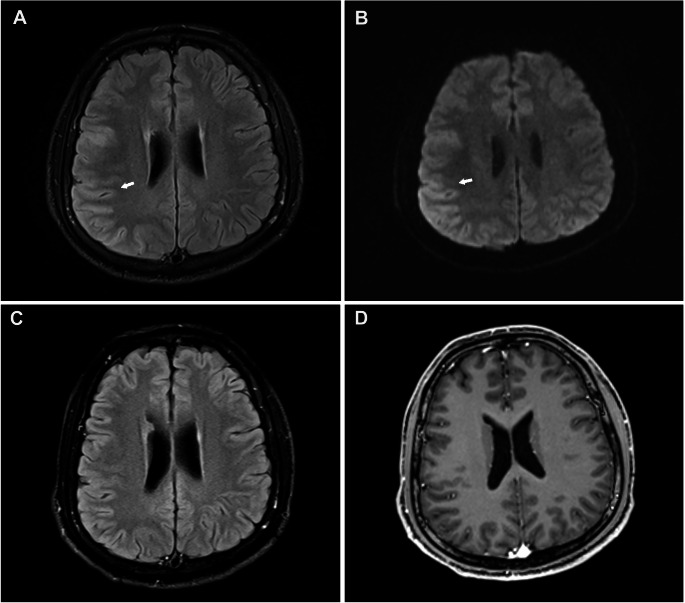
Brain magnetic resonance imaging. A and B Brain MRI shows T2/FLAIR and DWI signal hyperintensities involving multiple cortical areas of the right temporal, occipital and parietal lobes (arrow). C and D T2/FLAIR and contrast-enhanced MRI four months after symptom onset showed no specific abnormalities in the cerebral cortex
